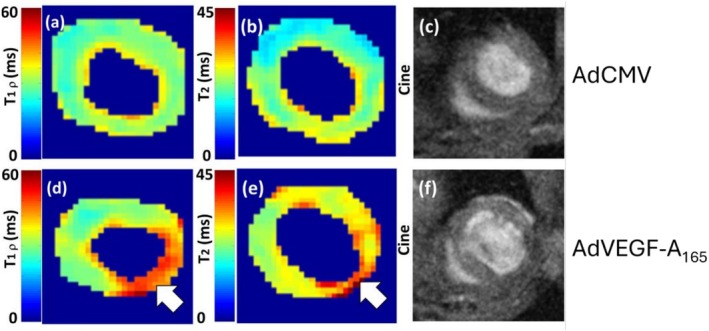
Relaxation time maps after 14 days of AdCMV and AdVEGF‐A_165_ gene transfers. (a) CW‐T_1ρ_ in AdCMV heart. (b) T_2_ in AdCMV heart. (c) Cine MR image of AdCMV heart. (d) CW‐T_1ρ_ in AdVEGF‐A_165_ heart. (e) T_2_ in AdVEGF‐A_165_ heart. (f) Cine MR image of AdVEGF‐A_165_ heart. White arrows indicate injection sites and the area of inflammation reaction.
